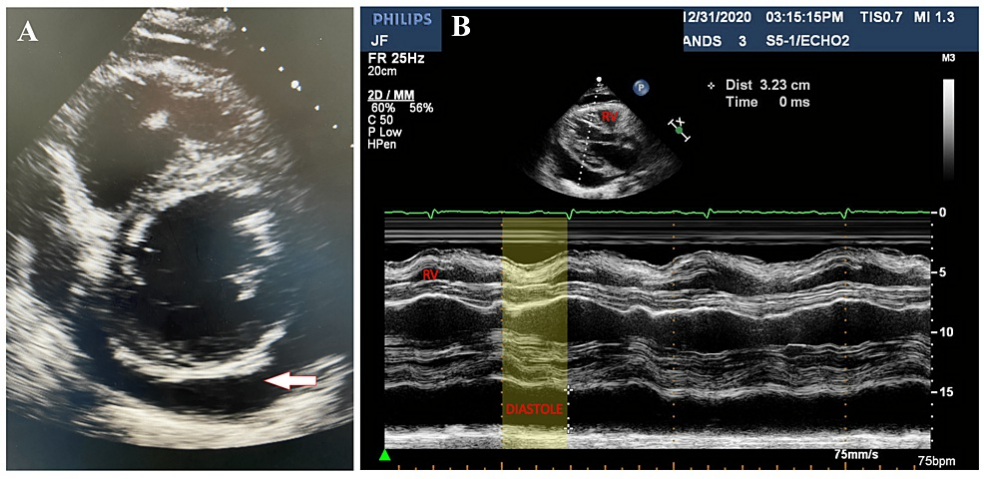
Echocardiography of pericardial effusion. (A) Parasternal short axis view of echocardiogram at the basal level of the left ventricle demonstrating an anterior/posterior view of the pericardial effusion (indicated by white arrow). (B) M-mode demonstrating diastolic collapse of the right ventricle (indicated by yellow shade) secondary to the pericardial effusion.
